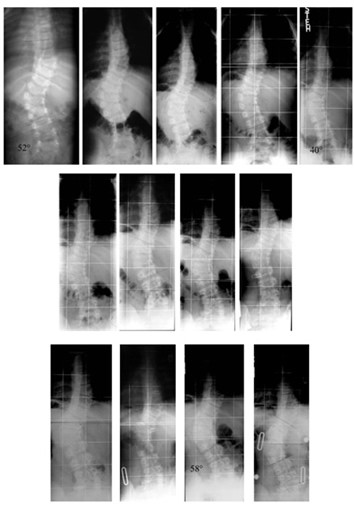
Congenital scoliosis due to failure of formation with a follow-up of 13 years to Risser 4 under conservative treatment with a brace. Patient with failure of formation and curve 52 degrees at the lumbar spine at the age of 18 months when brace treatment started. The three rows of x-rays show the complete radiological follow-up: - During the first five years of treatment (first row), the curve has been successfully reduced from 52° (first row left) to 46° (first row middle) at the age of 3.6 years to 40° (first row right) at the age of seven. - Between the age seven to 11 (second row of x-rays) there is no real difference in the follow-up x-rays and the in-brace x-ray (second row on the right) at the age of 11 shows no big correction. - At 13 years progression back to 50° appeared (third row on the left) and the new brace showed only little in-brace correction (third row middle left). The last brace was made at the age of nearly 16 years at Risser 4, when the curve had progressed to 58° after loss of compliance (third row on the middle right). In-brace x-ray showed no real correction in the mature boy (third row on the right).
